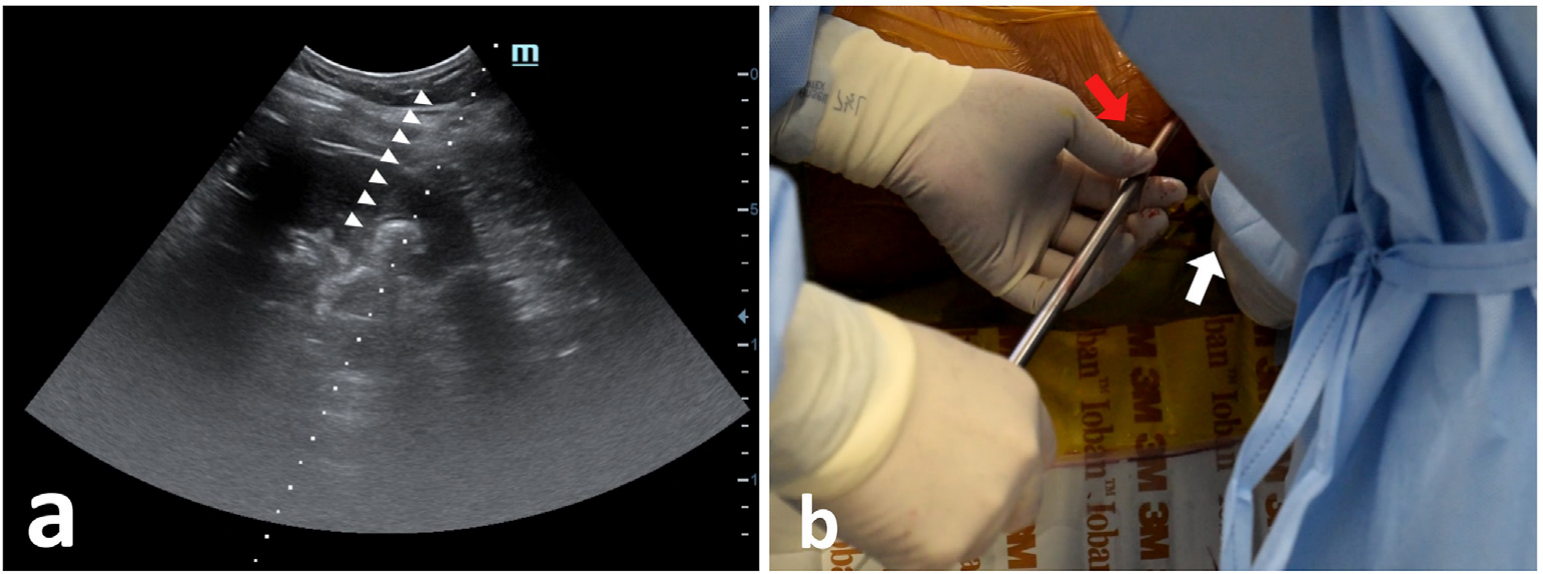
Ultrasound guided renal tract dilatation using alken metal dilator. (a) Visualisation of Alken metal dilator can clearly be seen through ultrasound imaging. (b) Alken metal dilator, held by operator, inserted serially (red arrow) up to 30 Fr guided by ultrasound visualization. Ultrasound probe (white arrow) held by the operator assistant.
